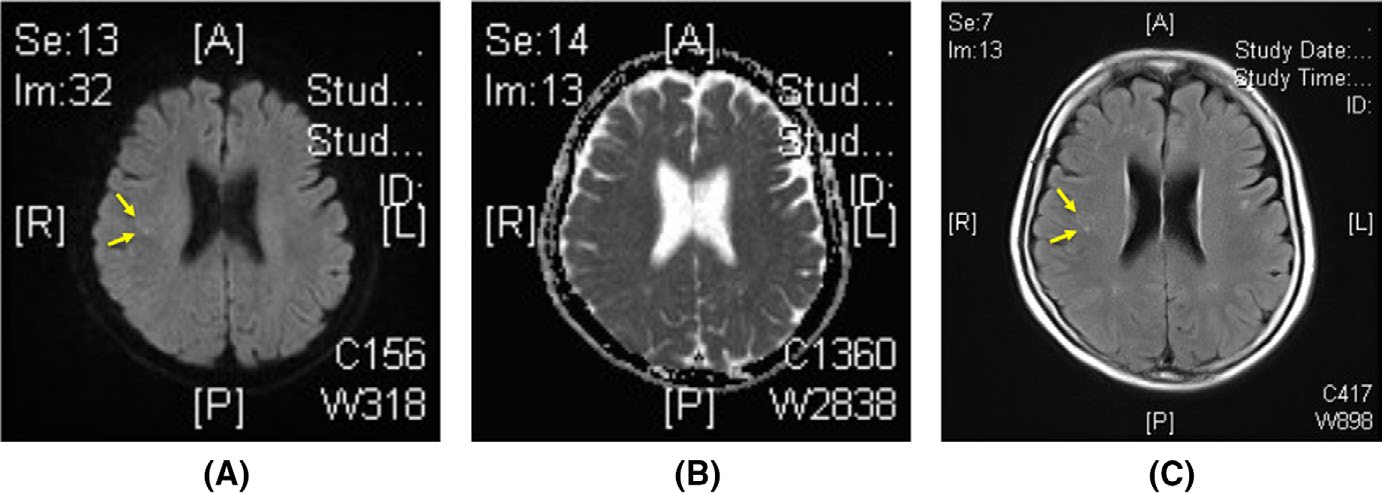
Brain magnetic resonance images (MRI) of a patient who underwent AF ablation and developed subacute silent stroke. (A) Diffusion‐weighted image (DWI), (B) Apparent diffusion coefficient (ADC) map, and (C) fluid‐attenuated inversion recovery (FLAIR) image. The patient complained of mild dizziness 3 weeks after the AF ablation. The brain MRI images reveal a round‐shaped lesion (arrows) in the right parietal lobe suggesting subacute stroke. No abnormal findings are observed in cerebellum or brain stem
